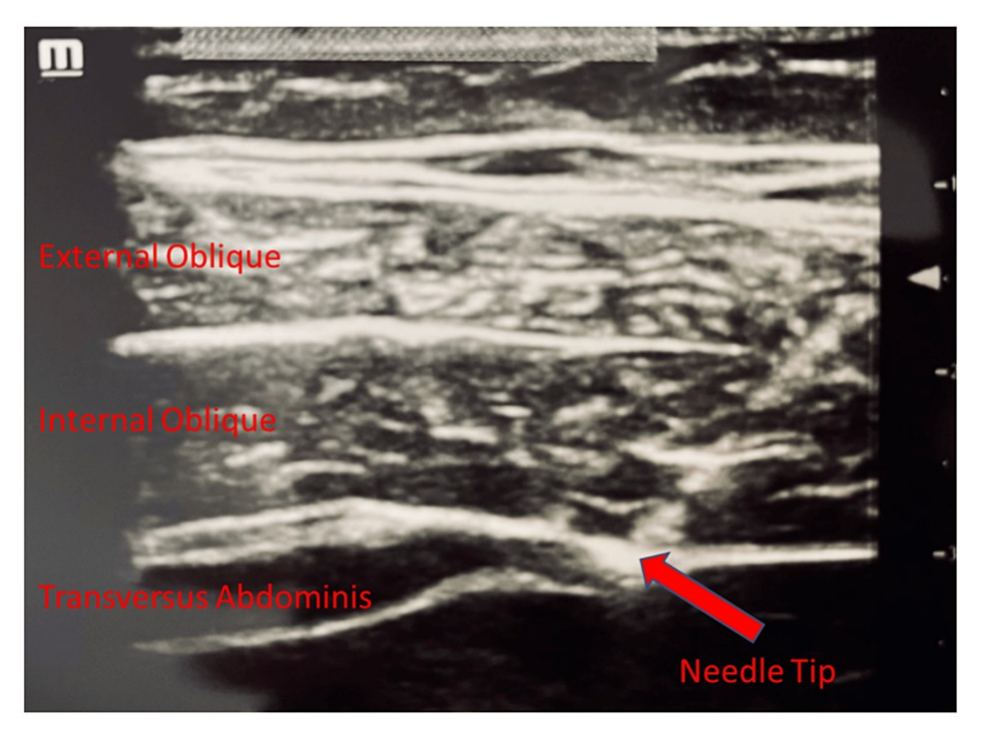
Ultrasound-Guided Quadratus Lumborum Block Using the QL1 Technique
